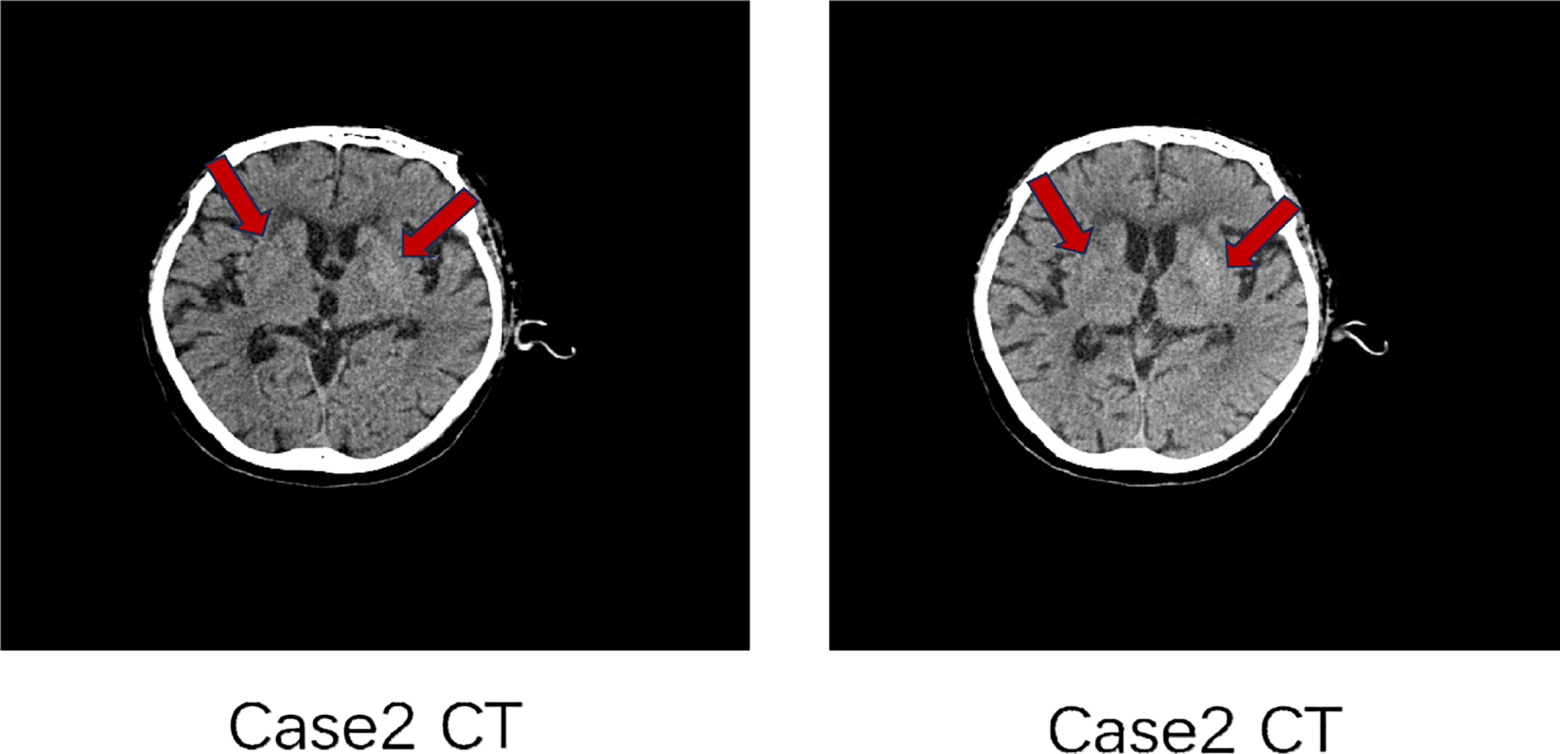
Imaging manifestation of case 2. CT, computed tomography.
